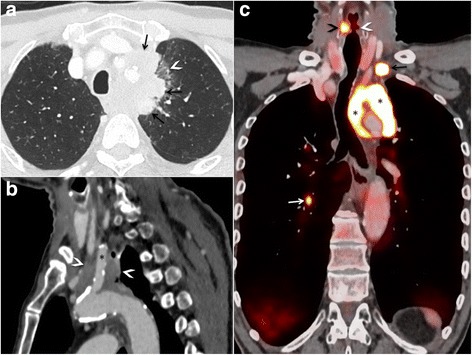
(a) Axial contrast-enhanced computed tomographic (CT) scan (lung window) depicts an upper left mediastinal mass (*arrows*) infiltrating the left upper lobe, which displays a “ground-glass opacity” (*arrowhead*). (b) Sagittal contrast-enhanced 2D reconstruction (soft tissue window) shows local tumoral extension (*arrowheads*) surrounding the aortic arch and origin of the left common carotid artery (*asterisk*). Both arteries are patent. (c) Coronal contrast-enhanced positron emission tomographic-CT scan reveals left upper lobe and mediastinal fludeoxyglucose (^18^F-FDG) uptake (*asterisks*). Hypermetabolic left supraclavicular lymph node (*black arrow*) and hypermetabolic pulmonary nodule in the right lower lobe (*white arrow*). Indirect signs of left recurrent laryngeal nerve palsy are present in terms of enlarged left laryngeal ventricle (*white arrowhead*) along with compensatory increased ^18^F-FDG uptake of the right vocal cord (*black arrowhead*)
